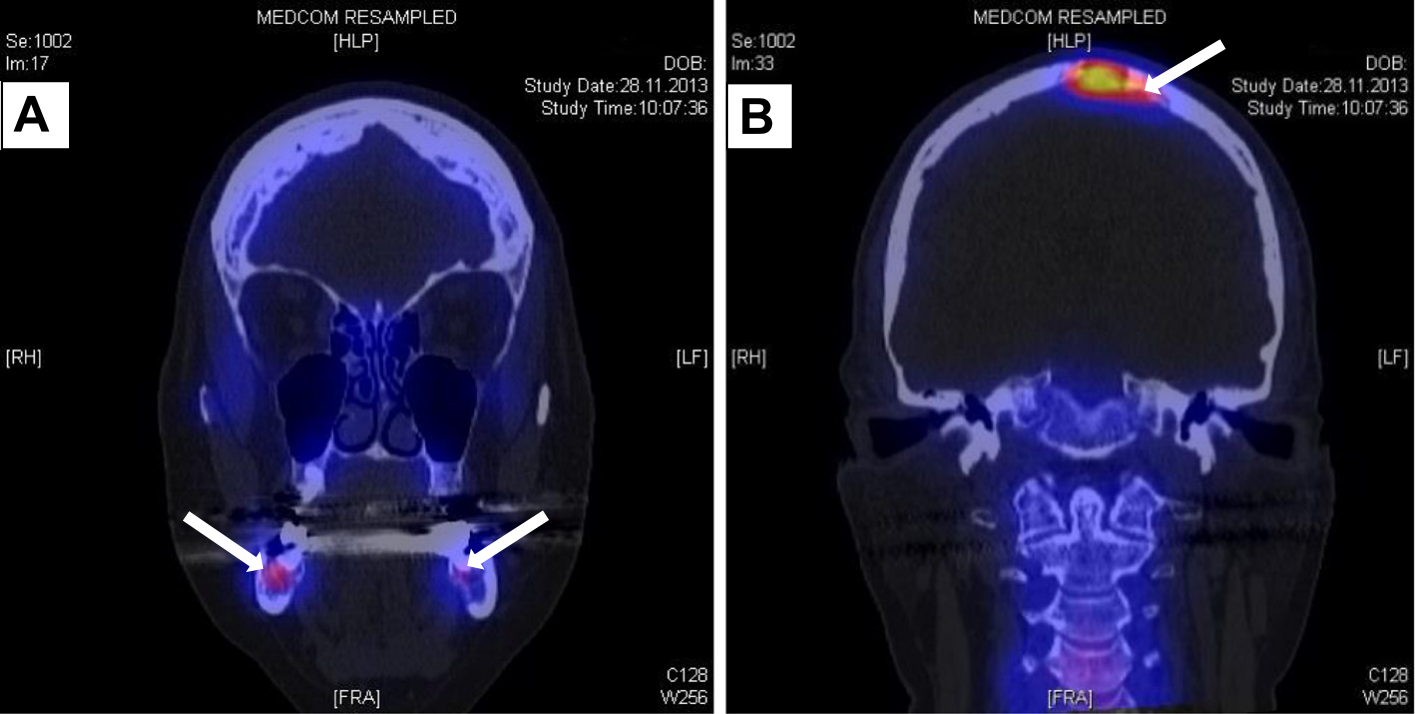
SPECT-CT imaging of the head. The coronal view presents increased bone metabolism in the area of both jaw angles a and in the area of the vertex b which is indicated with white arrows
